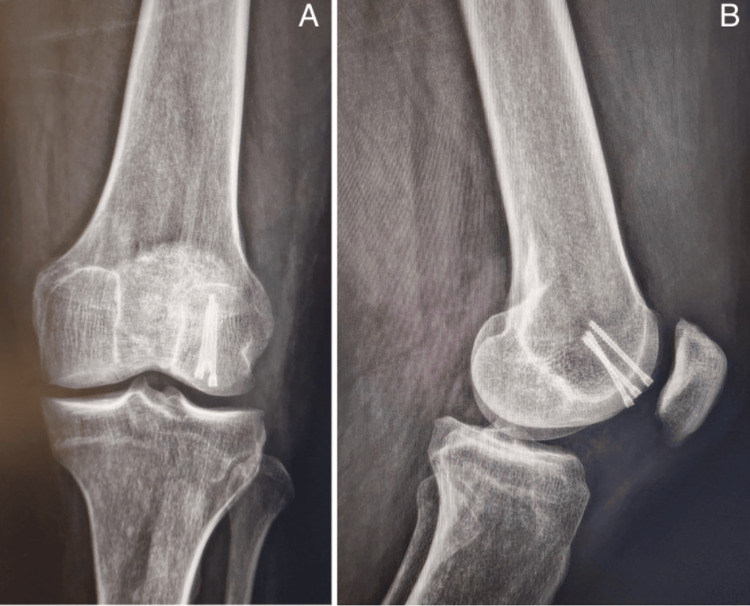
Follow-up X-ray at eight weeks post-intervention showing consolidation of the osteochondral fragment.
